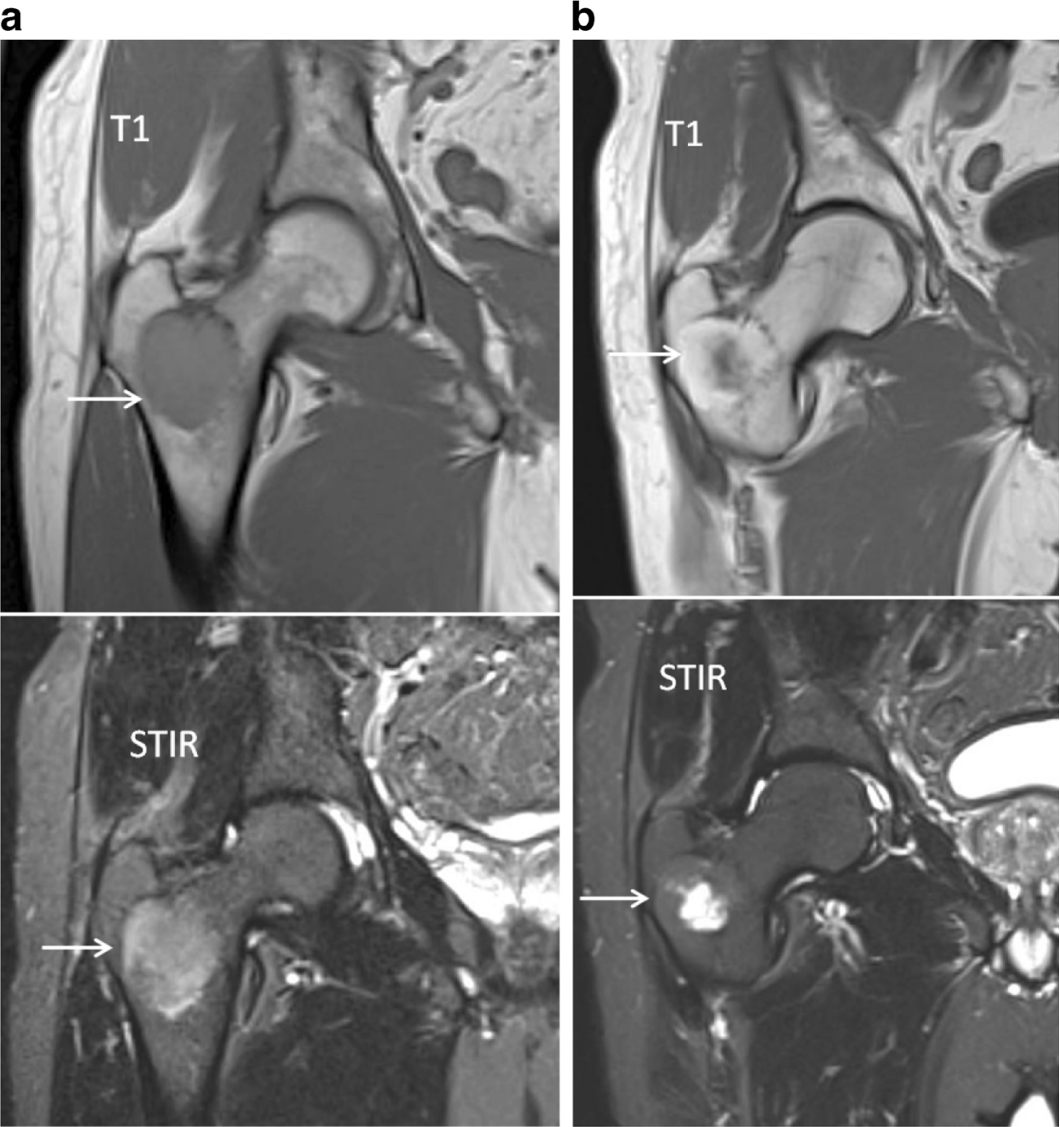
T1-weighted (*top*) and T2-weighted STIR (*bottom*) images of newly diagnosed myeloma patient a with diffuse infiltration of the bone marrow and a large focal lesion in the right intertrochanteric region (*arrows*). Five years after receiving systemic therapy and local irradiation on the right intertrochanteric region b there is an increase in signal intensity in the centre of the focal lesion on STIR images compatible with necrosis. Also remark the broad fatty halo surrounding the lesion. Both of these imaging findings indicate good response to therapy
